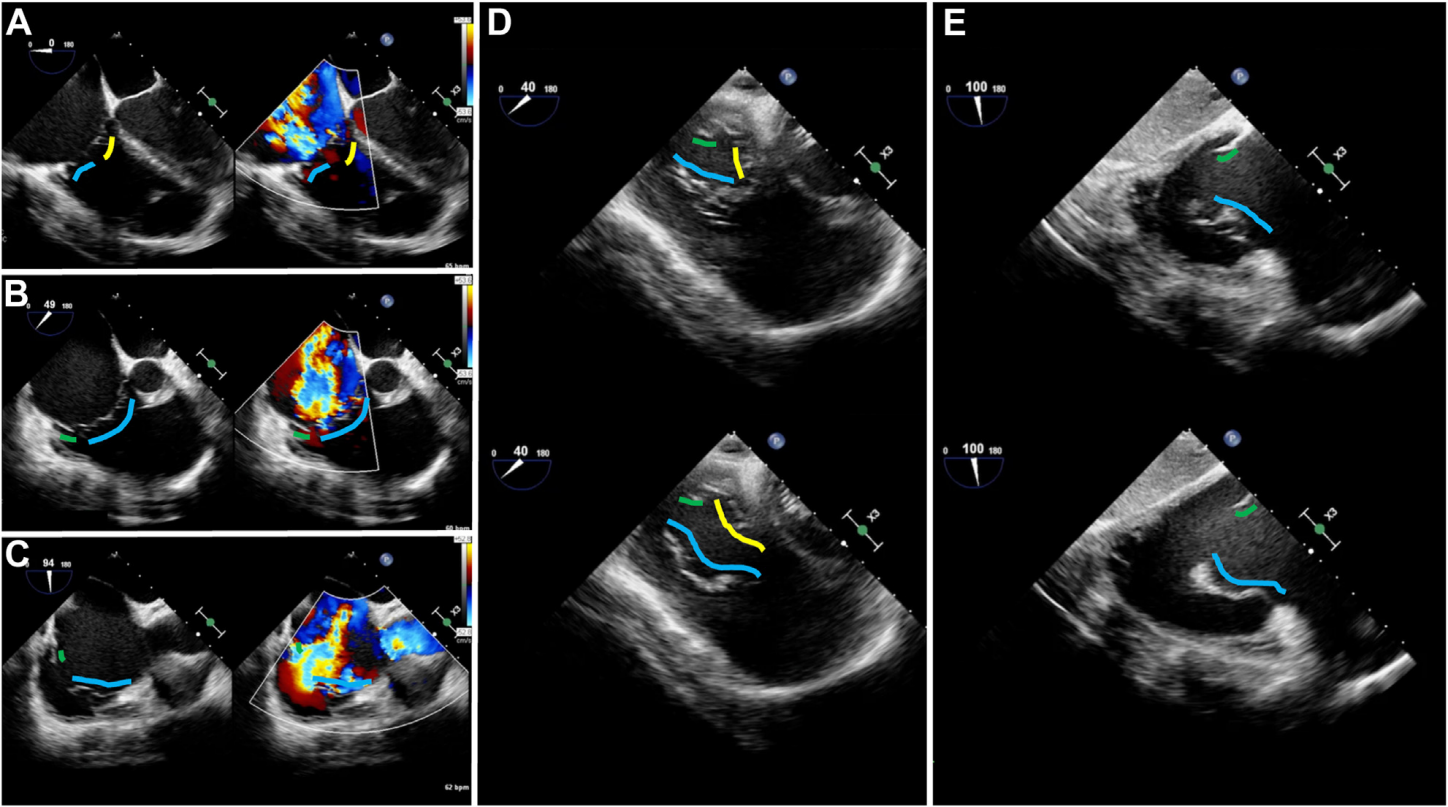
Two-dimensional TEE, midesophageal level, systolic views without (*left*) and with (*right*) color-flow Doppler in the (A) 4-chamber view (0°), (B) short-axis view (49°), and (C) oblique bicaval view (94°), demonstrates the morphology of the anterior (*blue*), septal (*yellow*), and posterior (*green*) TV leaflets. The posterior leaflet is tented and hypoplastic and there is severe TR. In the transgastric window, short-axis (40°) (D) and long-axis (100°) (E) views of the TV leaflets in systole (*top*) and diastole (*bottom*), the hypoplastic posterior leaflet contributing to malcoaptation is seen.
